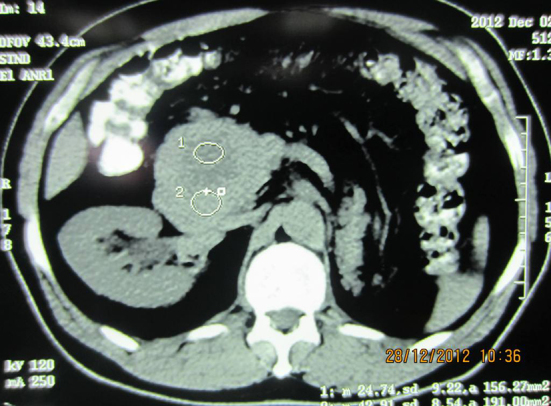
Abdominal CT scan shows pancreaticoduodenal mass and absence of right kidney.
